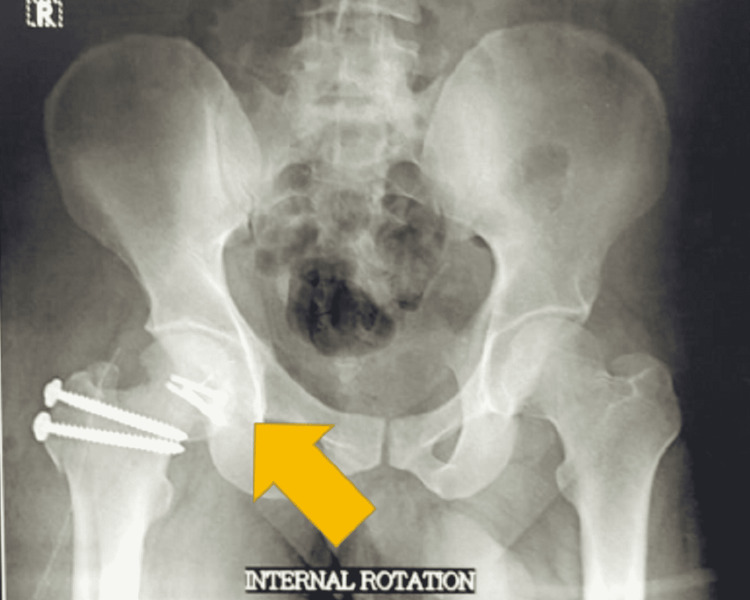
Internal rotation radiograph of pelvis anteroposterior view showing reduced Pipkin’s fracture at six months follow-up.
